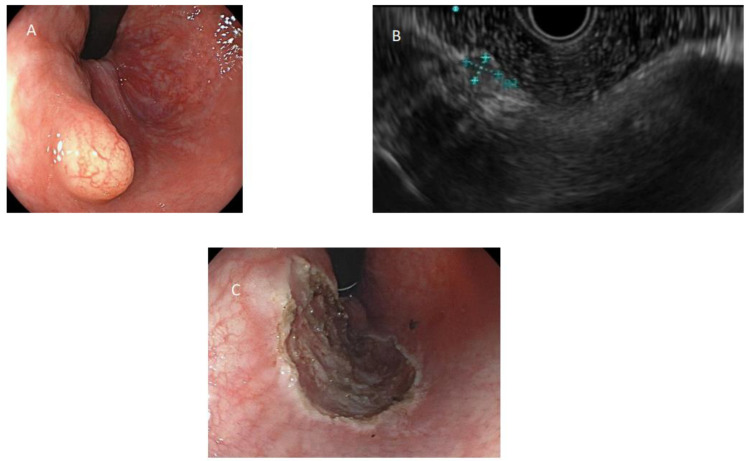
(A) Rectal neuroendocrine tumor with typical appearance of a yellowish sub-epithelial lesion, visualized in retroversion; (B) endoscopic ultrasound aspect of a rectal neuroendocrine tumor; (C) cutting eschar after removal using cap-assisted endoscopic mucosal resection.
